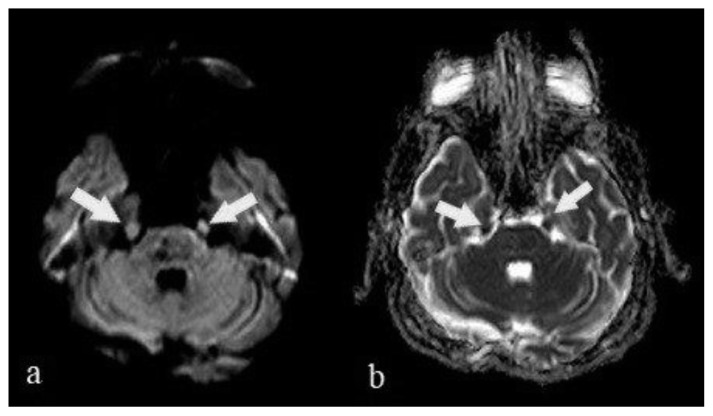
Bilateral trigeminal nerve thickening of a 69 years old man was revealed on DWI (a) and ADC (b) images (arrows).
